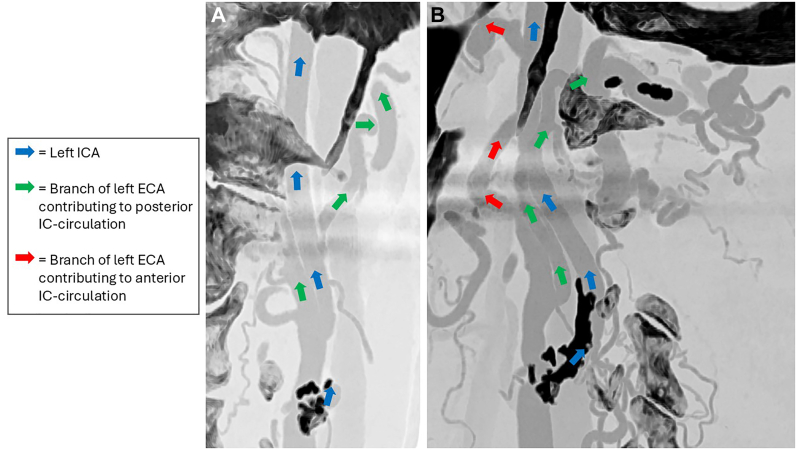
Computed tomography angiography (CTA) showcasing extensive collaterals from the left external carotid artery (*ECA*). (A) Frontal view. (B) Sagittal view. *IC*, Intracranial, *ICA*, internal carotid artery.
